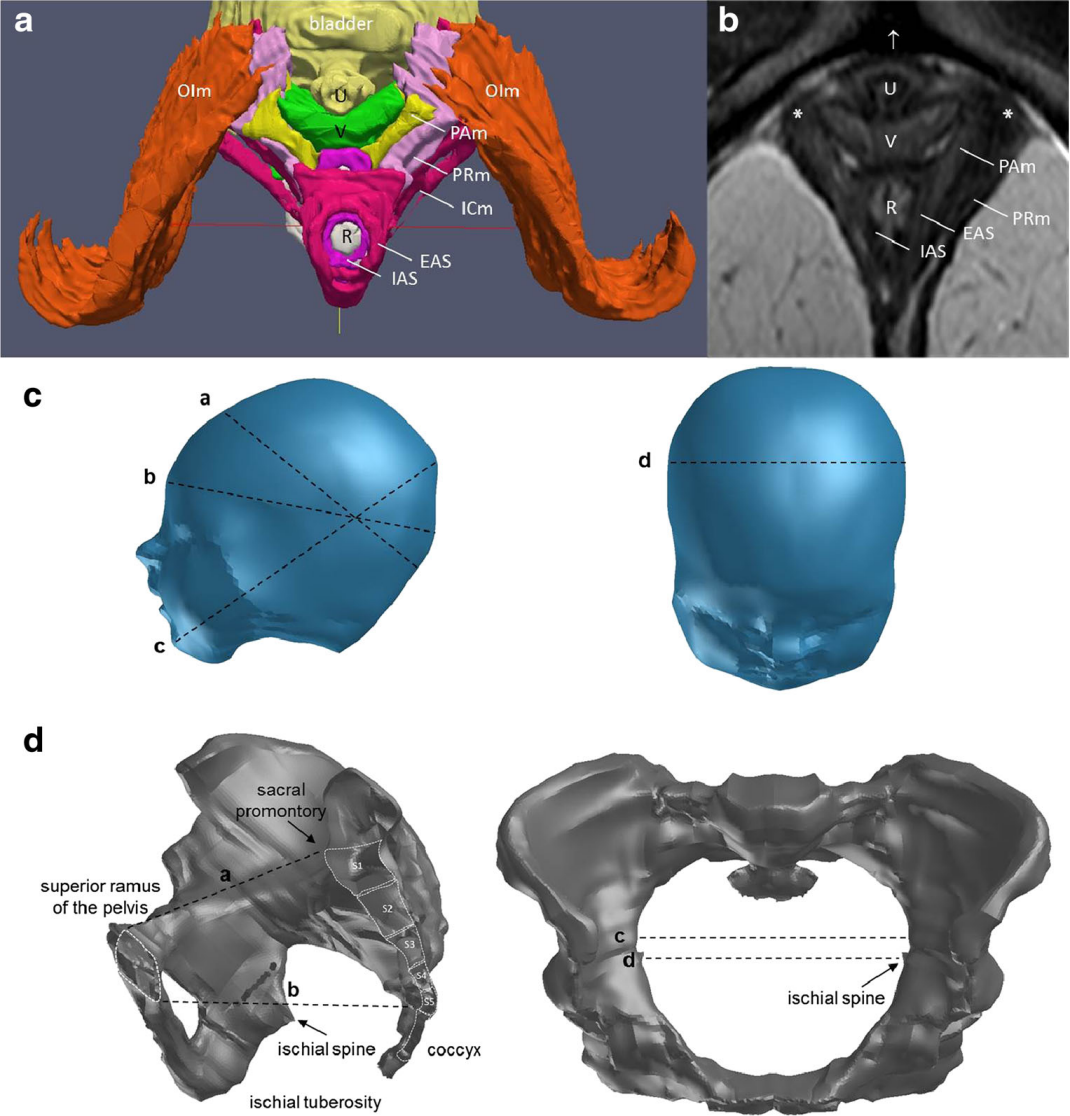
a View of the LAM muscle from below. Vulvar structures, perineal membrane, and bony pelvis are not visualized. Internal obturator muscle (*OIm*, *orange*), external anal sphincter (*EAS*, *light red*), internal anal sphincter (*IAS*, *dark purple*), puboanal muscle (*PAm*, *yellow*), puborectal muscle (*PRm*, *light purple*), iliococcygeus muscle (*ICm*, *dark red*), urethra (*U*, *light yellow*), vagina (*V*, *green*), and rectum (*R*, *gray*). b Relevant axial MRI shows the urethra (*U*), vagina (*V*), rectum (*R*), and muscles of the levator ani. Note the attachment of the levator ani muscle (*asterisk*) to the pubic bone. The arcuate pubic ligament is shown (*arrow*). c The principal dimensions of the fetal head: *a* suboccipitobregmatic dimension = 100.3 mm, *b* occipitofrontal dimension = 105.7 mm, *c* occipitomental dimension = 131.9 mm, *d* biparietal diameter = 93.7 mm. d The principal dimensions of the female bony pelvis. Lateral view of the pelvic bones: *a* pelvic inlet = 113.4 mm, and *b* pelvic outlet = 145.1 mm. Axial view demonstrating *c* transverse diameter of the pelvic inlet = 148.8 mm and the *d* interspinous diameter = 146.7 mm
